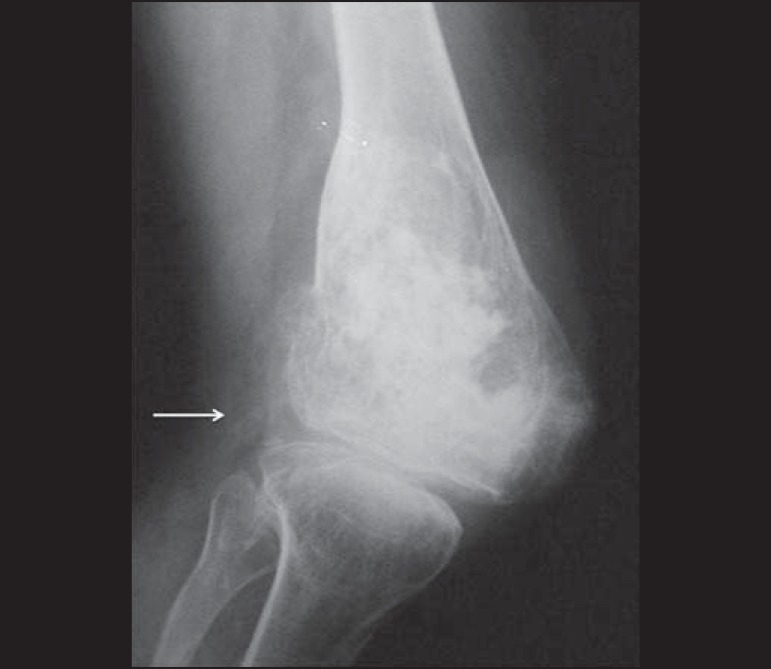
Radiograph of an adult with knee pain showing an aggressive, mixed
